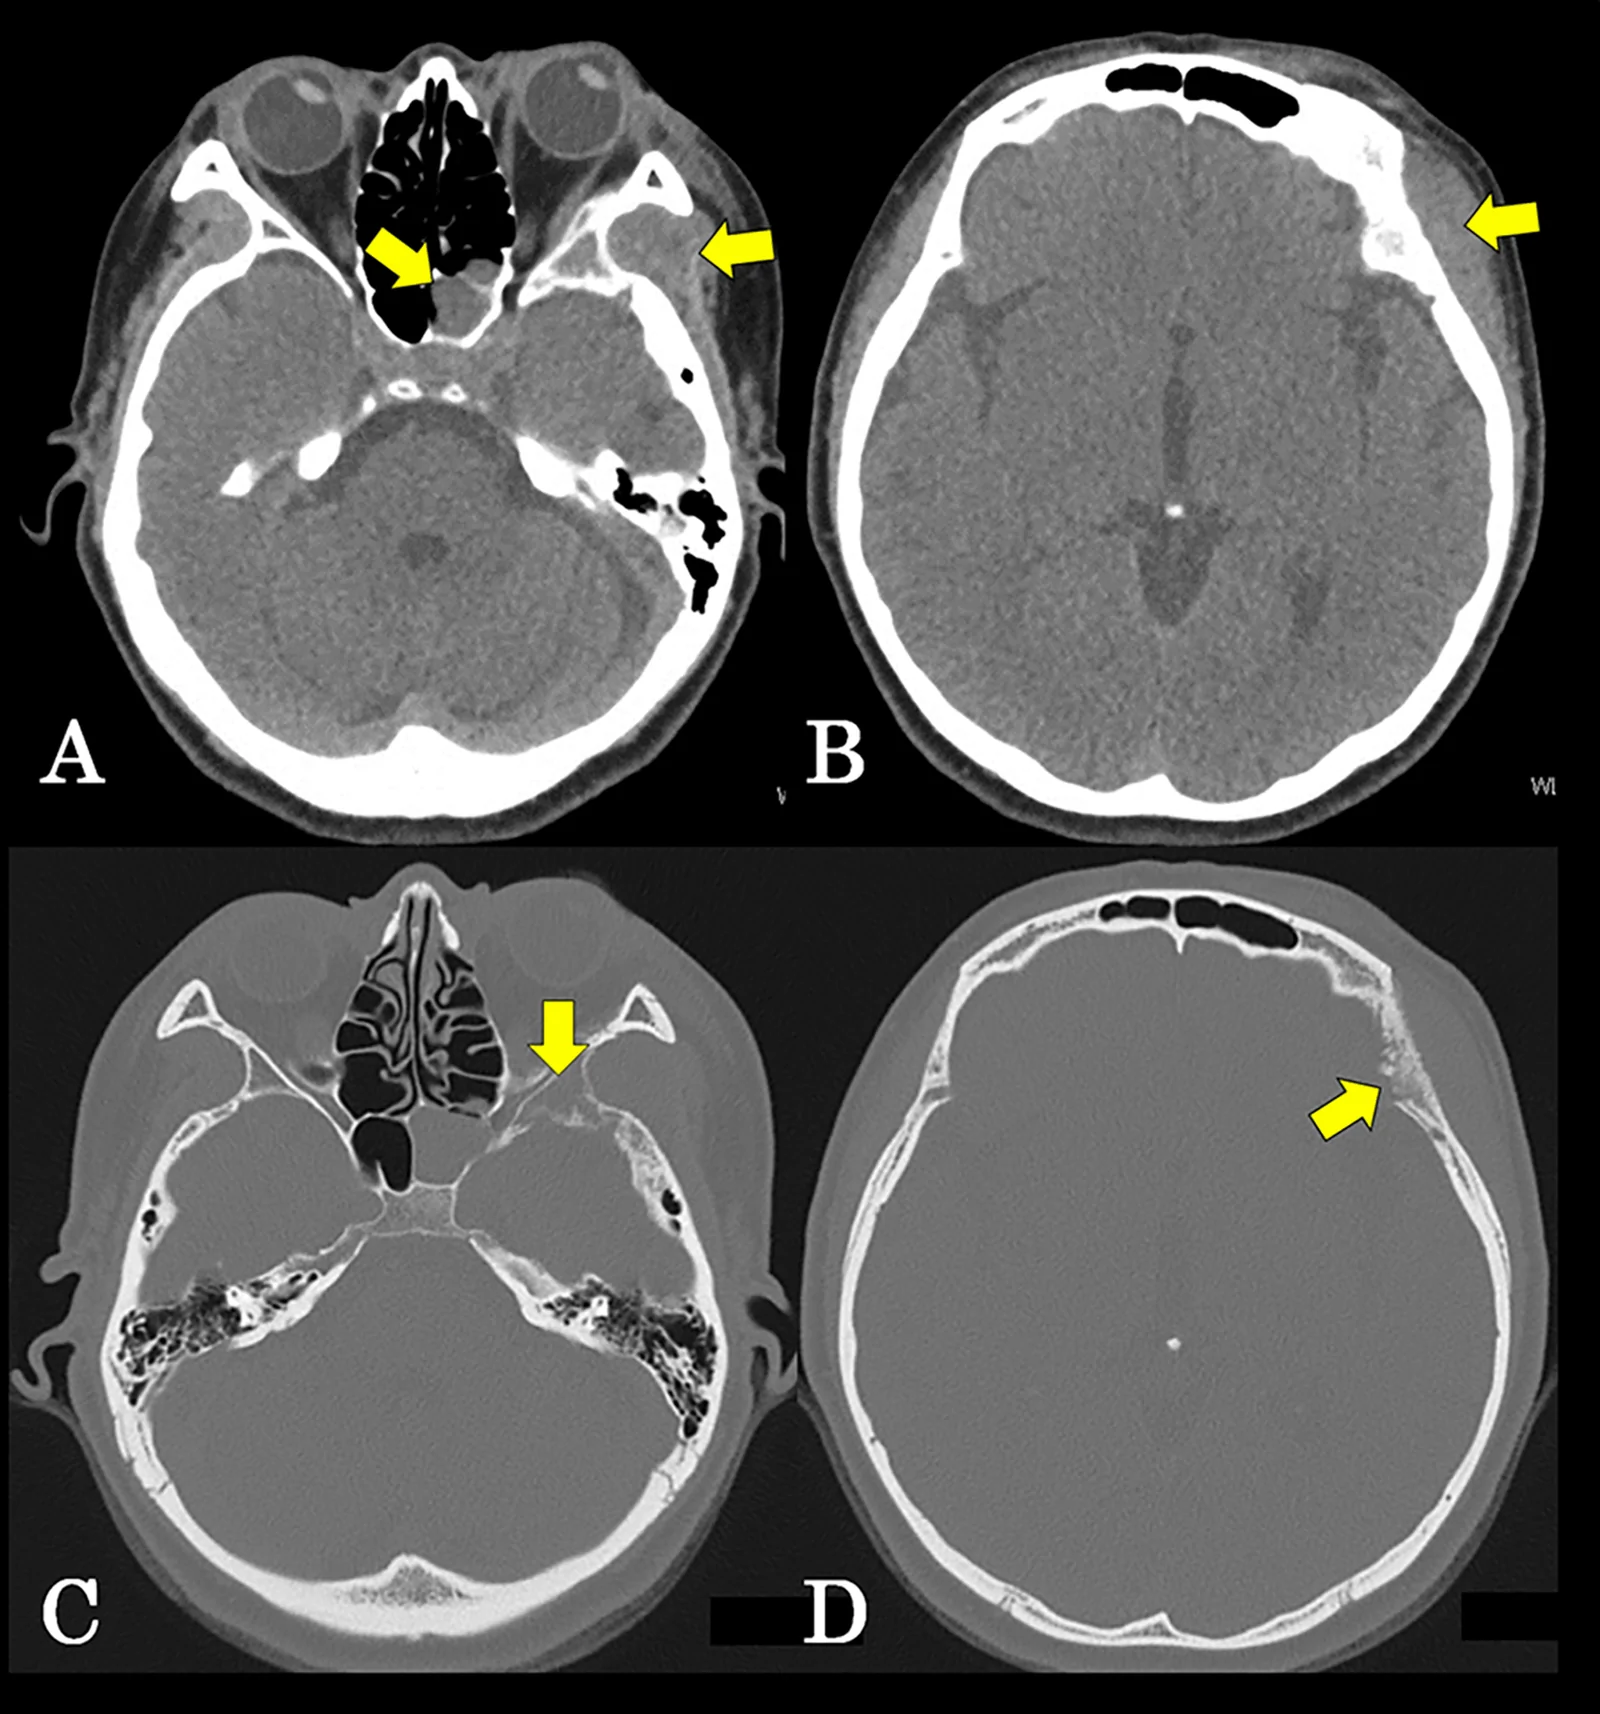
Computed tomography findings Axial CT images at (A) the level of the sphenoid sinus demonstrating a mass lesion occupying the sphenoid sinus and (B) the level of the orbit and temporal region showing tumor extension to the lateral orbit and temporal region. Arrows indicate the tumor. (C, D) Bone window CT images demonstrating mild thinning and remodeling of the left sphenoid bone and part of the left frontal bone without evidence of overt bone destruction. Arrows indicate bone abnormalities.
